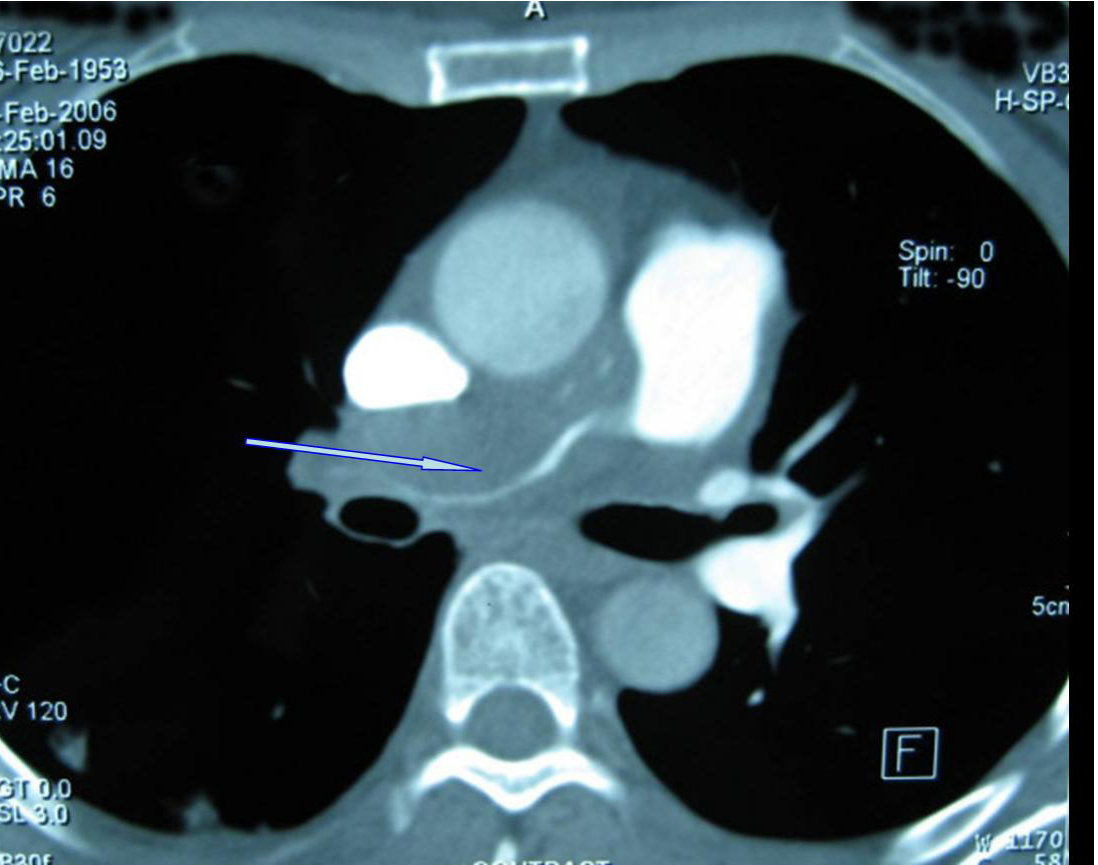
A computed tomography scan of the chest shows the main pulmonary artery was obstructed circumferentially.
